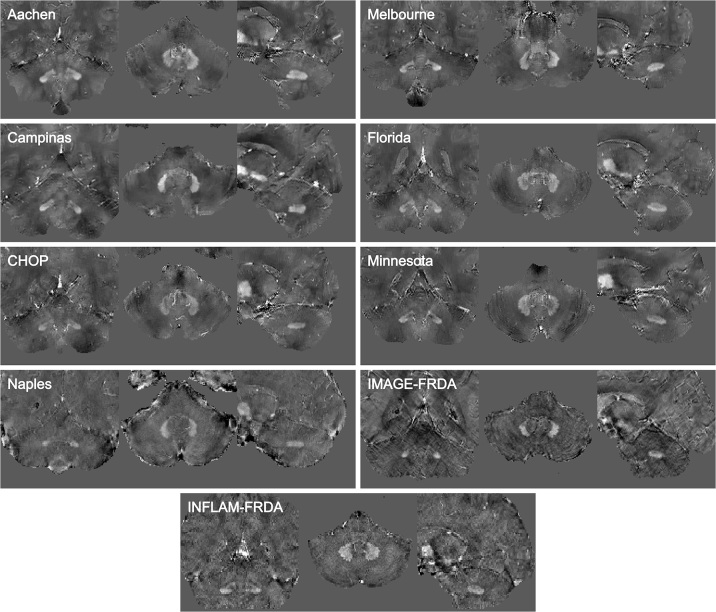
MR images show samples for each dataset. The images were randomly
